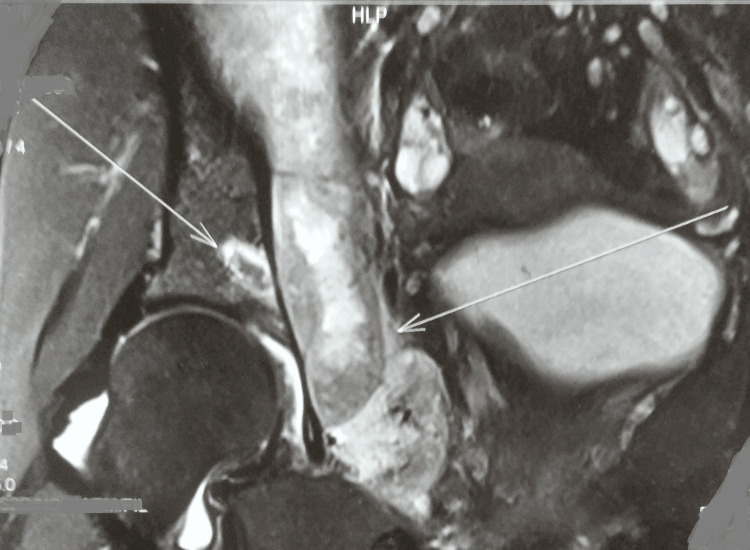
MRI of the right hip showing the lesions and abscesses MRI: magnetic resonance imaging
